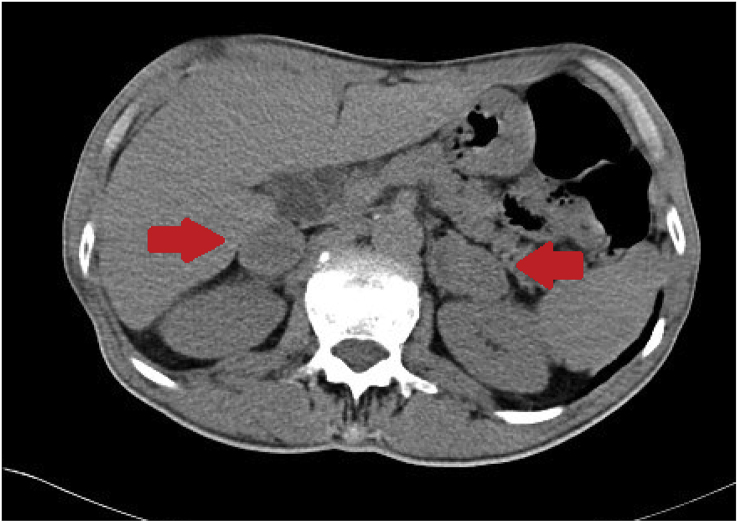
(Case 1) Day +3 Abdominal CT. Bilateral enlargement of adrenal glands bilaterally with some central hypodense areas (red arrows). (For interpretation of the references to colour in this figure legend, the reader is referred to the Web version of this article.)
